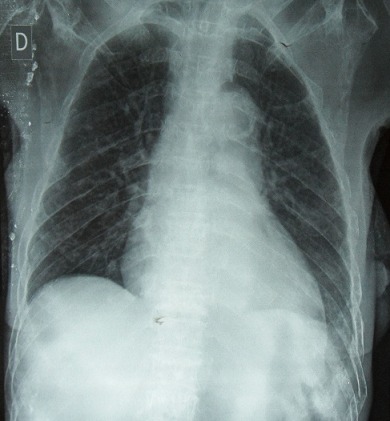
Radiographie thoracique de face montrant des fractures multi-étagées de la 6^ème^, 7^ème^ et 8^ème^ côte droite
